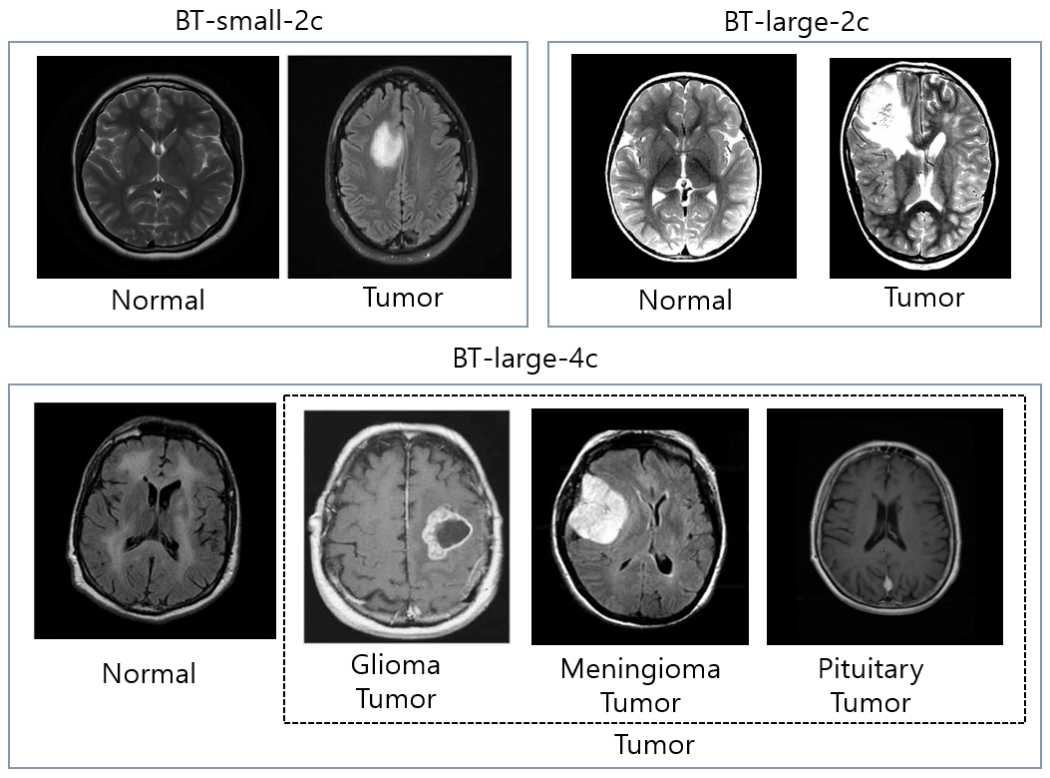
The examples of brain MR images in BT-small-2c, BT-large-2c, and BT-large-4c datasets.
